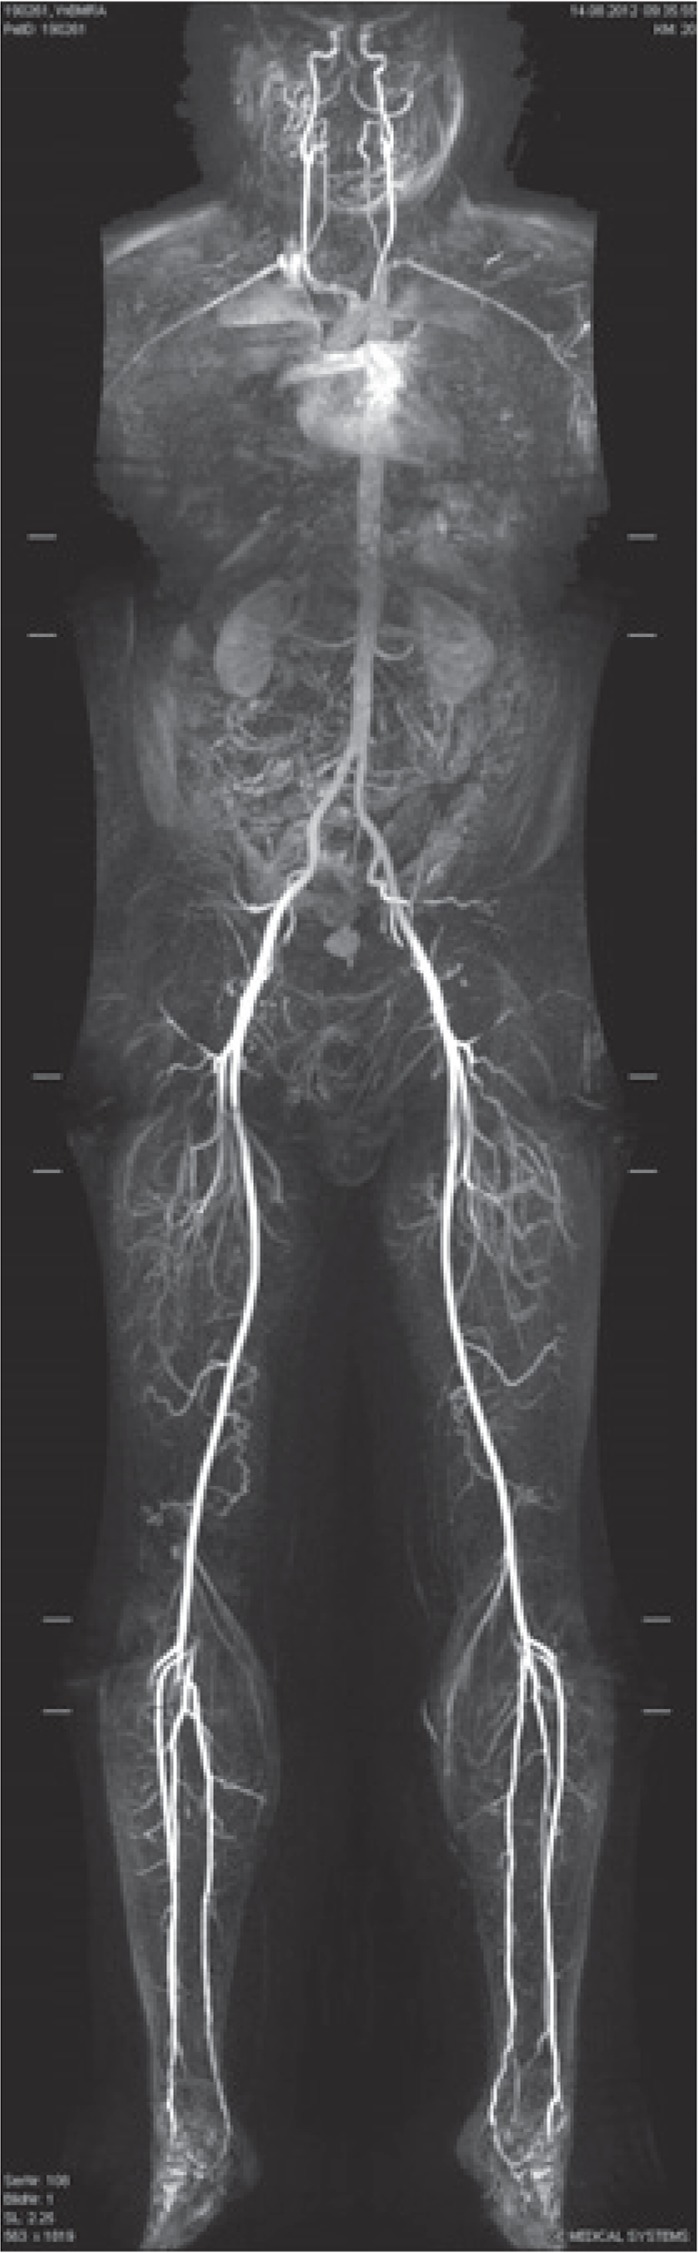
Whole-body MRA acquired on a dedicated whole-body MRI system and matrix coils with a single injection of contrast agent in an asymptomatic male 52-year-old patient, coronal reconstruction, pasted image.
